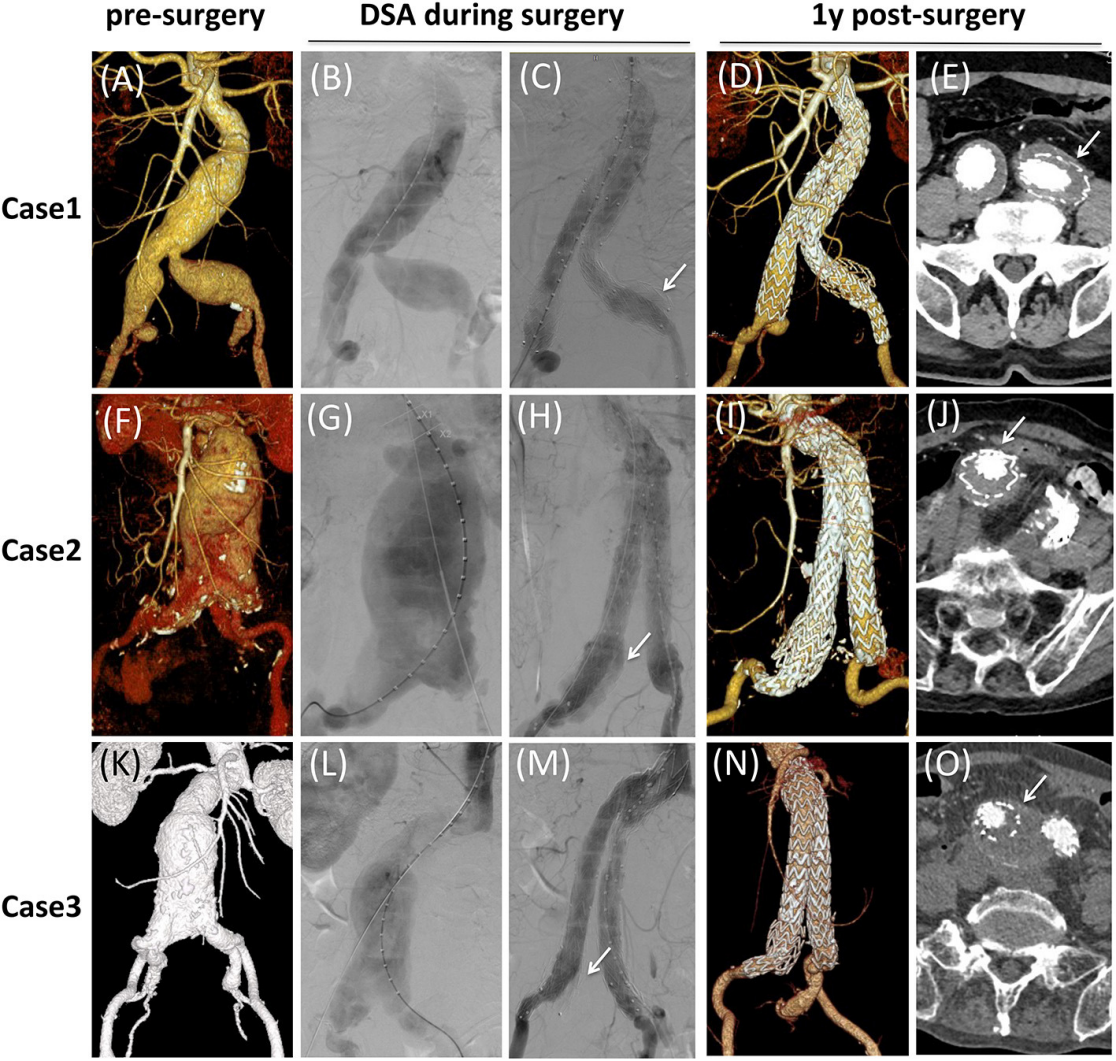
Typical cases of CST for aorto-iliac aneurysms. (A,F,K) Pre-surgical CTA 3D reconstruction. (B,G,L) DSA of aneurysms. (C,H,M) DSA after CST procedure. The white arrow indicates reflux of IIA was contained between the skirt graft and the extended iliac limb. (D,I,N) One-year follow-up CTA 3D reconstruction. (E,J,O) Transverse section of 1-year follow-up CTA. The white arrow indicates thrombosis formed in space between skirt graft and extended iliac limb.
